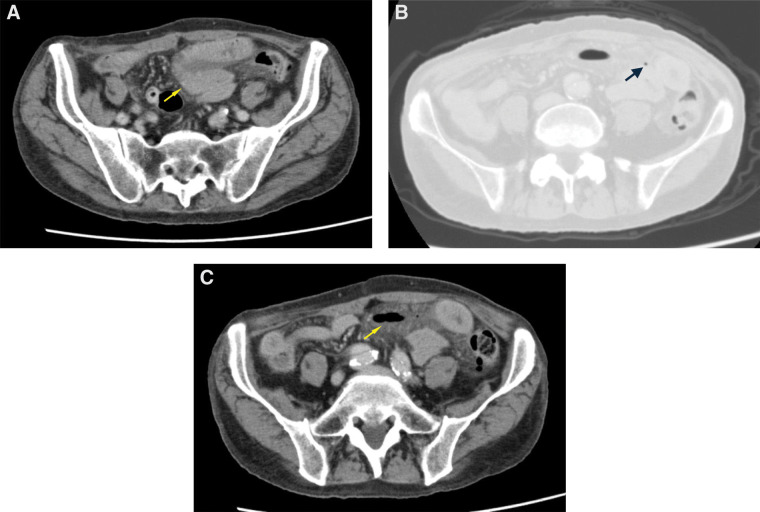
Noncontrast CT findings. Noncontrast CT image obtained at the time of initial presentation demonstrating localized wall thickening of the pelvic jejunum (arrow) (A) and an extremely small amount of free air adjacent to the affected small bowel (arrow) (B). Repeat noncontrast CT image obtained the following day, revealing a moderate amount of free air with intraperitoneal fluid leakage (arrow) (C).
